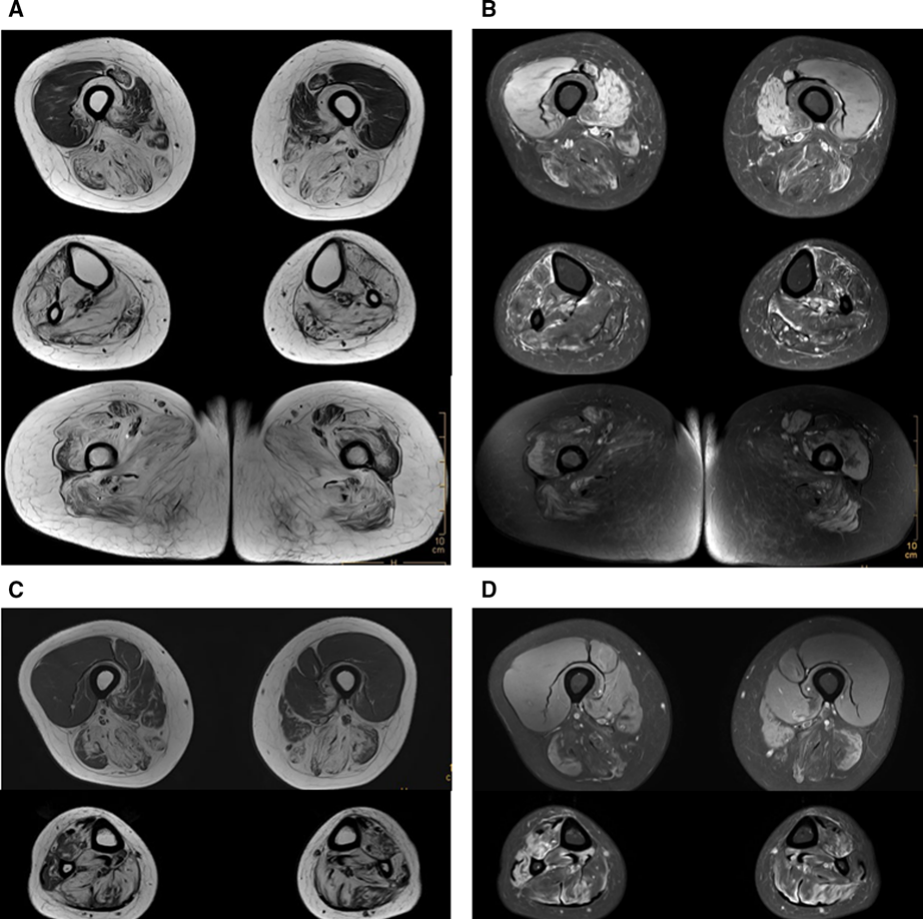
MRI in patients (II:3 and II:5) revealed serious fatty replacement in posterior and medial compartments of the thigh muscles and almost all lower leg muscles, which also presented in the buttock muscles of the patient (II:3). (A and B), axial T1‐weighted and axial PDw SPAIR MRI of thigh, lower leg and buttock muscles in patient (II:3). (C and D), axial T1‐weighted and axial PDw SPAIR MRI of thigh and lower leg muscles in patient (II:5)
